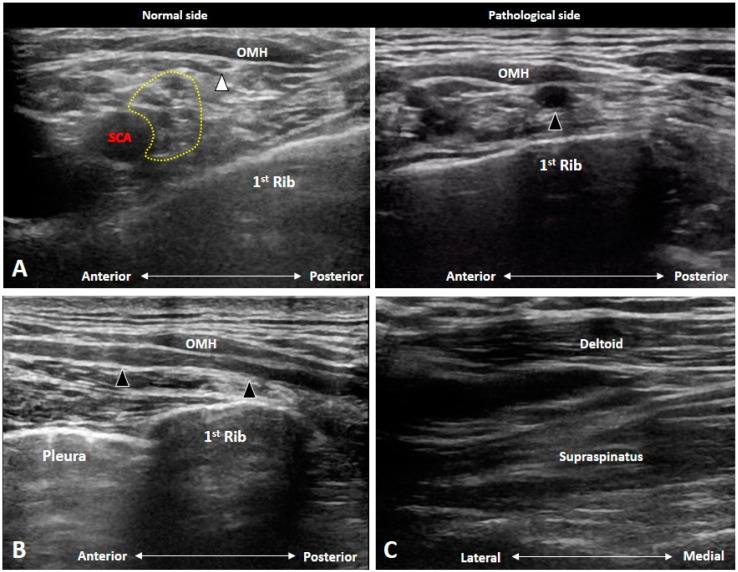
Ultrasound imaging of the suprascapular nerve (black arrowheads) in a patient with Parsonage-Turner syndrome (A). The normal nerve (white arrowhead) on the contralateral side (A). The enlarged suprascapular nerve in the long-axis view (B), atrophy and fat infiltration of the supraspinatus and infraspinatus muscles (C) are also seen. OMH: omohyoid muscle; SCA: subclavian artery. The yellow dashed area encircles the brachial plexus.
